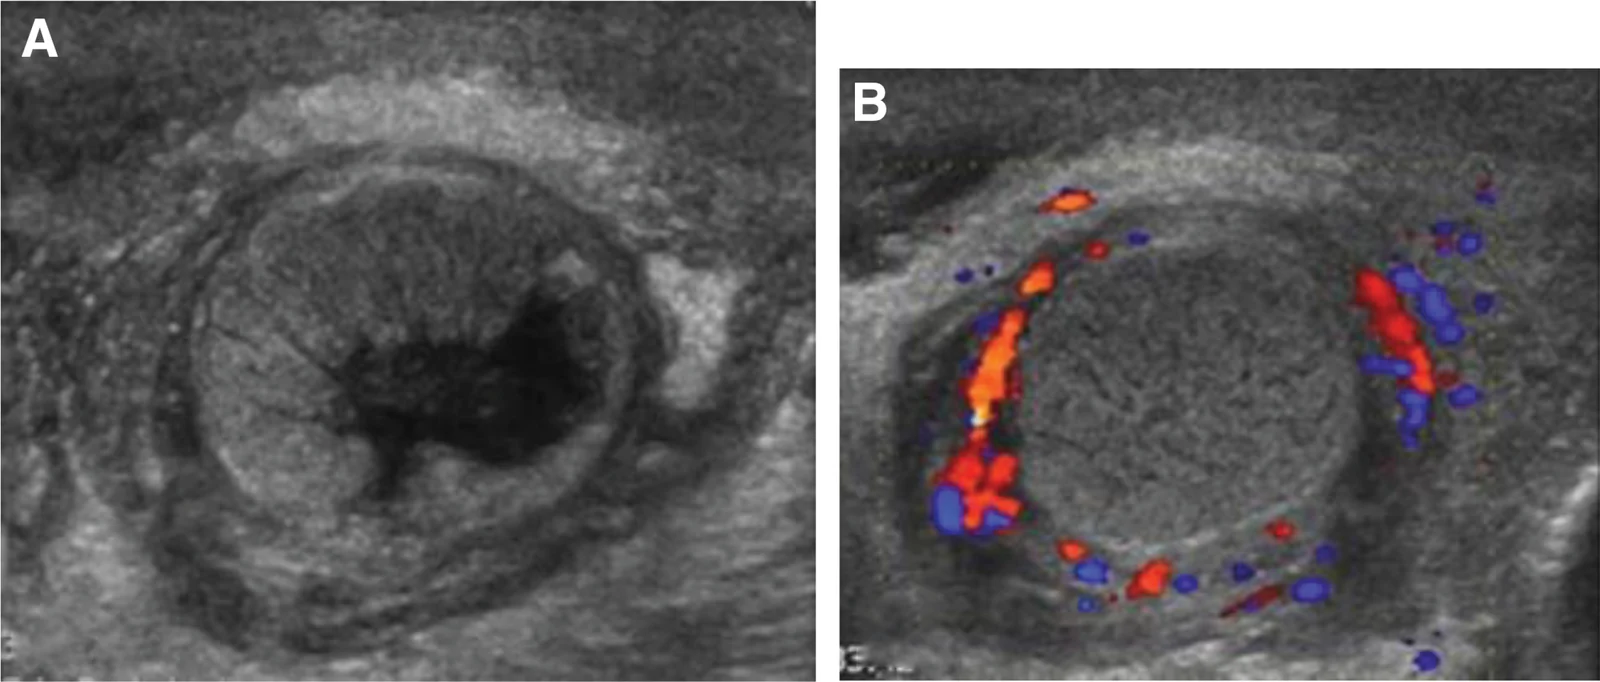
(A) Scrotal ultrasound demonstrated an enlarged testis with a markedly heterogeneous echotexture and absence of intratesticular blood flow on Color Doppler, consistent with neonatal extravaginal testicular torsion. (B) Color Doppler ultrasound revealed complete absence of intratesticular blood flow in the affected testis, confirming acute ischemia secondary to neonatal extravaginal testicular torsion.
